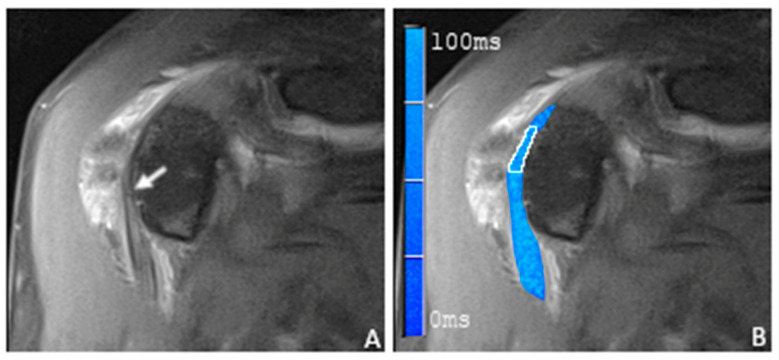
(A) A coronal proton-density-weighted fat-saturated magnetic resonance image of a 57-year-old man with longitudinal hyperintensity of the sulcal and the partially depicted extraarticular portion of the LBT with a markedly increased tendon diameter (arrow). (B) A merged image of the proton-density-weighted images and the corresponding color-coded T2 maps with the placed ROI in the sulcal portion of the LBT (white frame). Note the elevated average T2 mapping value of 52.8 ms. During the arthroscopy, a longitudinal partial tear was found.
